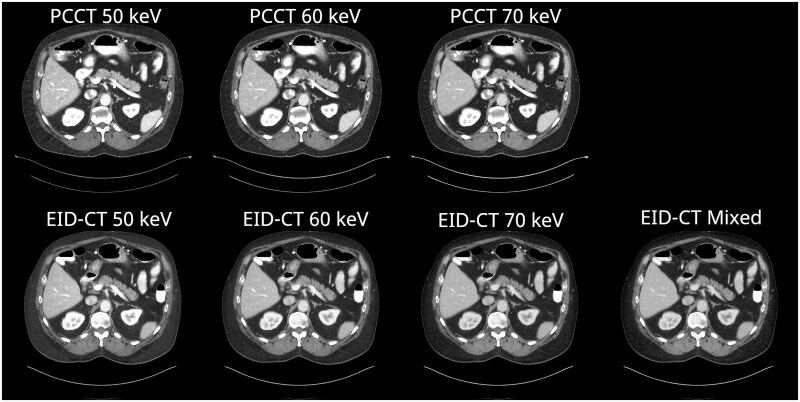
An example case (age 64, male) with all the image types included in the study. The display window and level for each image were individually adjusted to make the image appearing at the same grayscale. The radiation output (CTDIvol) for the EID-CT scan was 21.8 and 12.8 mGy for PCCT (41% dose reduction).
